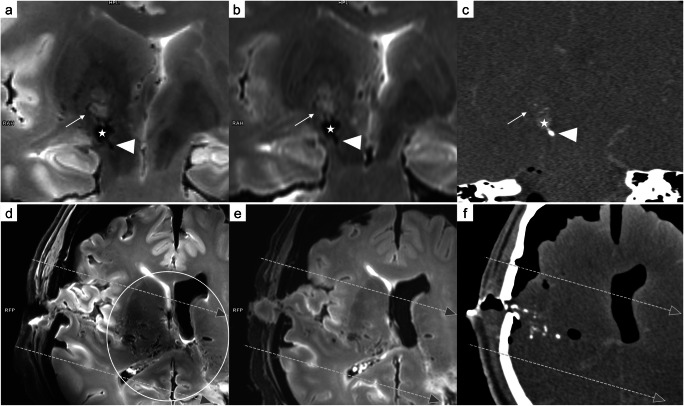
7 T T_2_-weighted images (a and d), 3 T T_2_-weighted images (b and e), and CT reconstruction (c and f). In a cross-section of the bullet path in case 1 (a-c: coronal view), soft tissue damage (arrow) was detected beyond the wound tract (asterisk). Differentiation between blood and bone fragments (arrowhead) was barely possible based on only the T_2_-weighted sequences. Furthermore, the 7 T images delineated numerous cavitation injuries in the region of the thalamus (circle) along the trajectory (dashed arrows)
